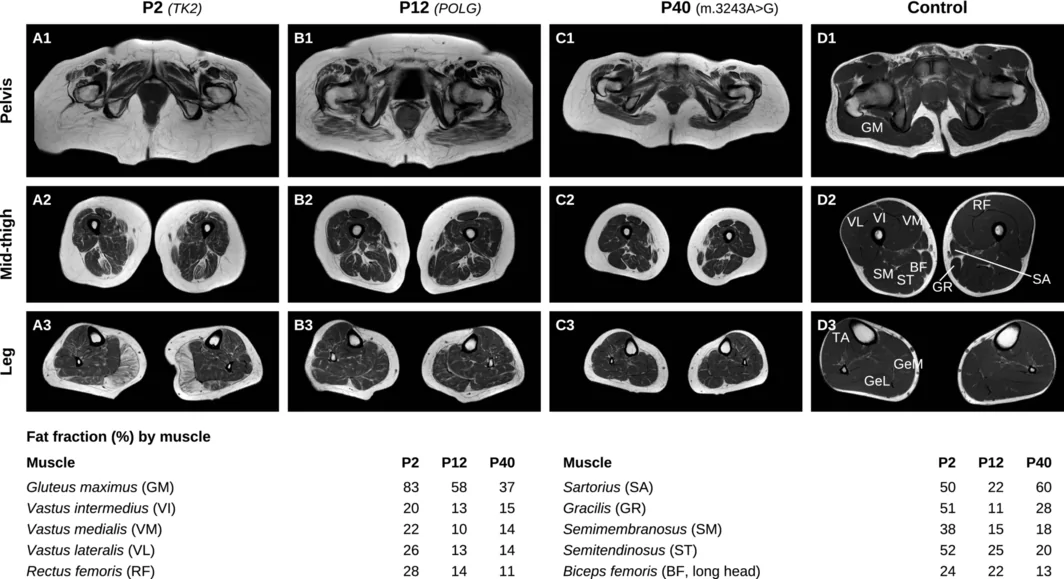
Axial muscle MRI at three anatomical levels in three patients and an unaffected control. Columns correspond to individual participants: (A) P2, *TK2*; (B) P12, *POLG*; (C) P40, m.3243A > G; (D) unaffected control. Rows correspond to the three standardised levels: (1) pelvis, (2) mid‐thigh, (3) leg. Images are axial T1‐weighted. Muscles are labelled on the control panels and abbreviated as follows: GM, *gluteus maximus*; VI, *vastus intermedius*; VM, *vastus medialis*; VL, *vastus lateralis*; RF, *rectus femoris*; SA, *sartorius*; GR, *gracilis*; SM, *semimembranosus*; ST, *semitendinosus*; BF, *biceps femoris* (long head); TA, *tibialis anterior*; GeM, *gastrocnemius medialis*; GeL, *gastrocnemius lateralis*. The leg muscles labelled in D3 are shown for anatomical orientation only and were not quantified. The table gives the fat fraction (%) of the 10 pelvic‐girdle and thigh muscles quantified in each patient.
